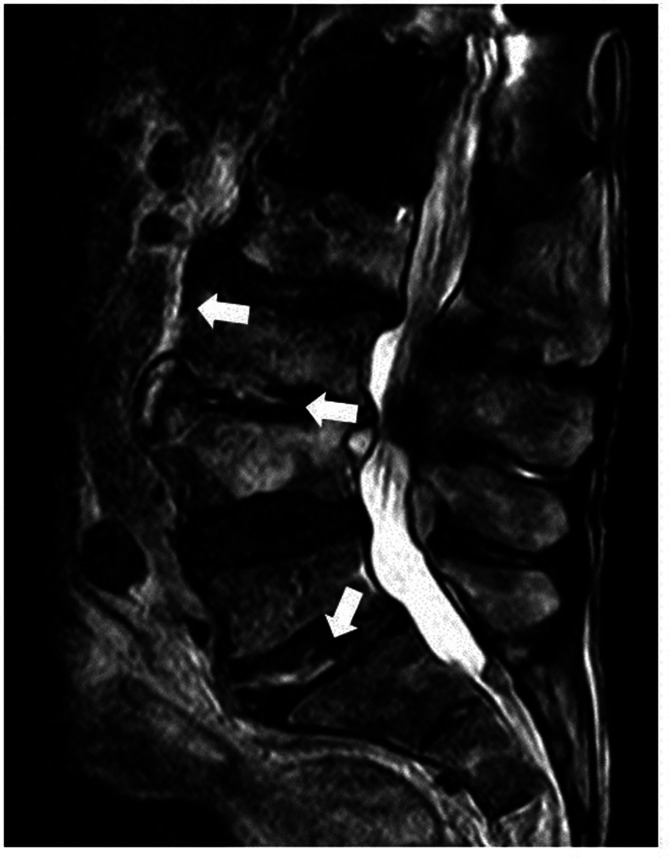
T2-weighted sagittal magnetic resonance imaging (MRI) scan of the lumbosacral spine. Hyperintense signals within the L3/L4 and the L5/S1 disc space and praevertebral L3 represent fluid accumulations.
